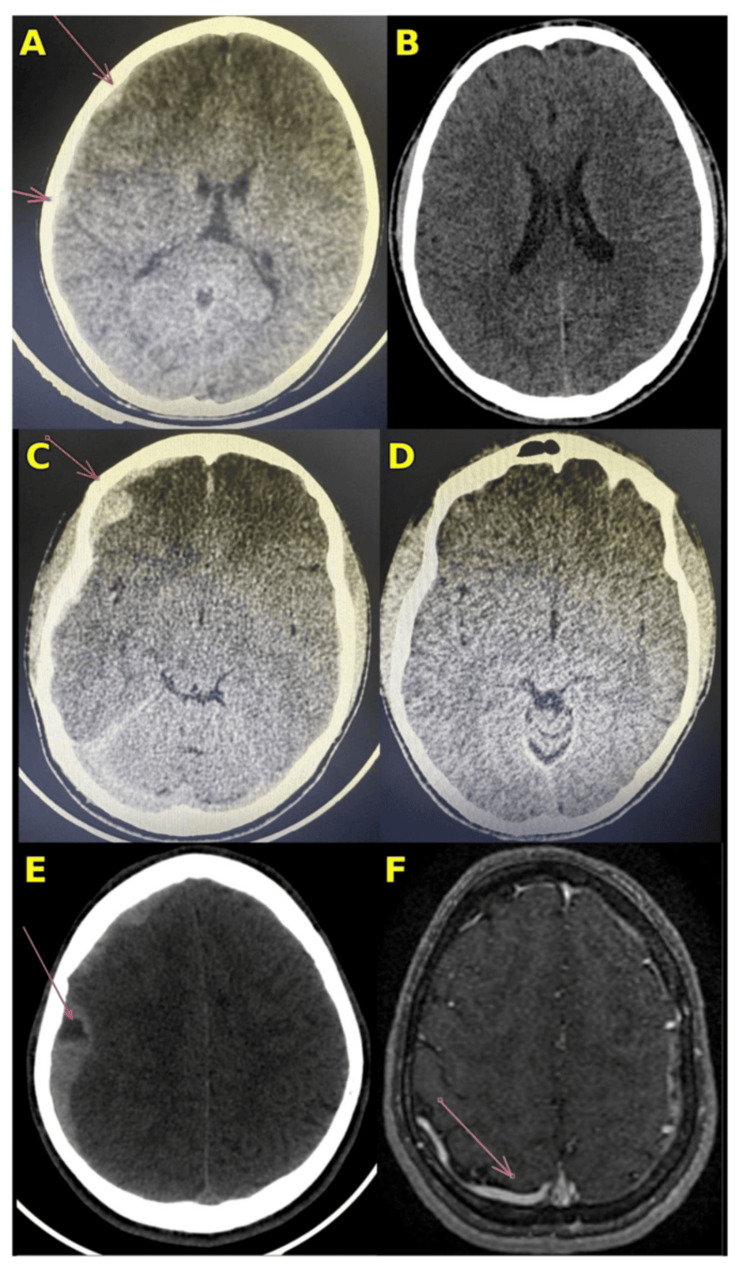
Neuroimaging findings across three episodes of recurrent strictly right-sided extra-axial intracranial hemorrhage and MRI/MRA features suggestive of a dural arteriovenous fistula. (A) Axial non-contrast brain CT at age 14 showing a right frontoparietotemporal epidural hematoma (orange and pink arrows) without significant midline shift. (B) Follow-up axial non-contrast CT one month later demonstrating complete radiologic resolution. (C) Axial non-contrast CT at age 17 years and 3 months showing a right frontotemporal subdural hematoma associated with a right frontal epidural hematoma (orange arrow) and mass effect with an approximately 5-mm leftward midline shift. (D) Follow-up axial non-contrast CT one month later demonstrating complete resolution. (E) Axial non-contrast CT at age 18 years and 1 month showing a heterogeneous right frontotemporoparietal subdural hematoma (pink arrow) with and an approximately 5-mm leftward midline shift. (F) Post-contrast axial T1-weighted MR angiography demonstrating an ectatic right parietal cortical venous structure (pink arrow) draining into the superior sagittal sinus, raising suspicion for arteriovenous shunting and a dural arteriovenous fistula.
